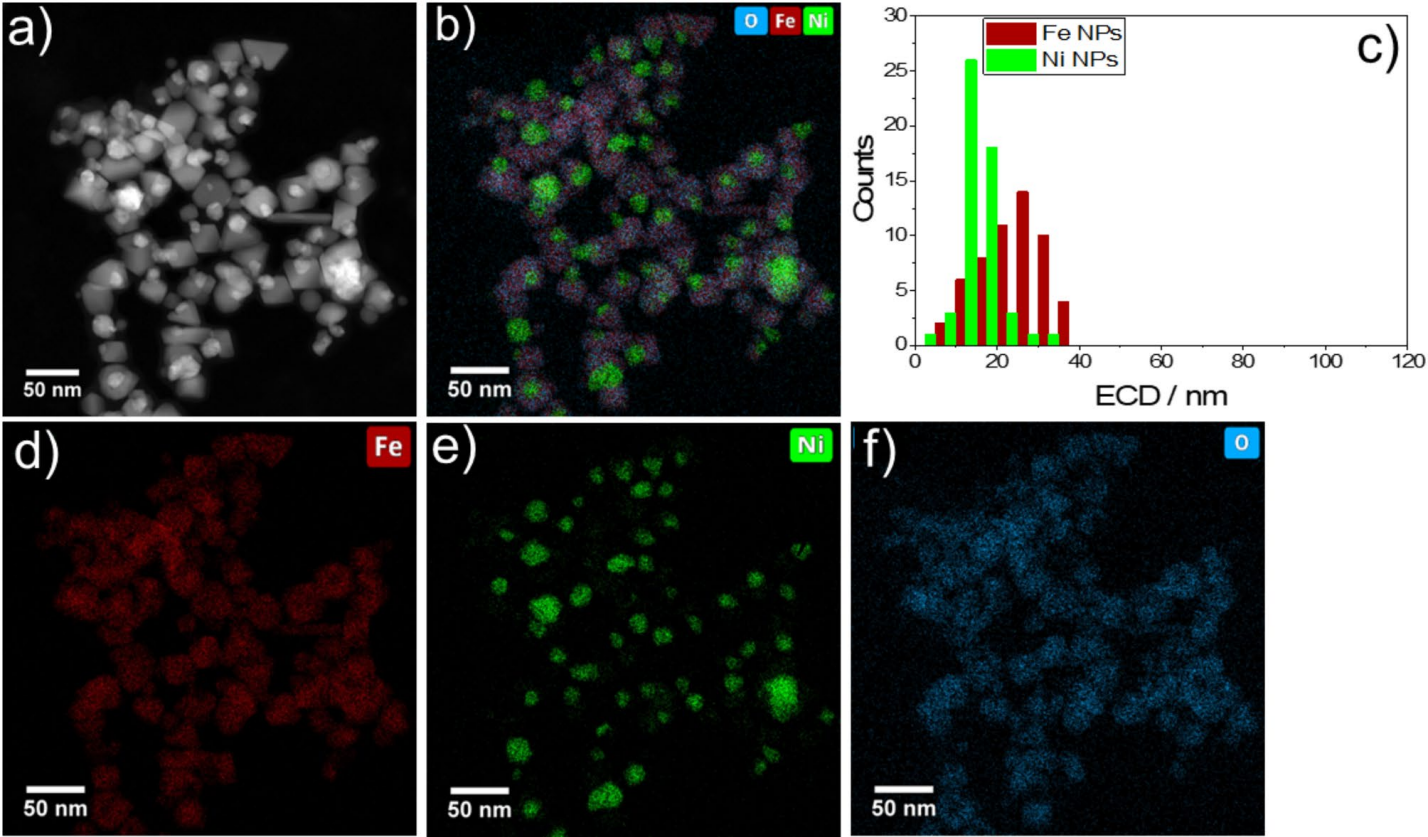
Fe: Ni = 3:1 NPs: (a) HAADF-STEM image, (b) EDS elemental mapping of O K, Fe K and Ni K X-ray lines, (c) Histogram of the size distribution of Fe and Ni NPs as detected by EDS in (b), (d) separate EDS elemental mappings of Fe (d), Ni (e), and O (f).
